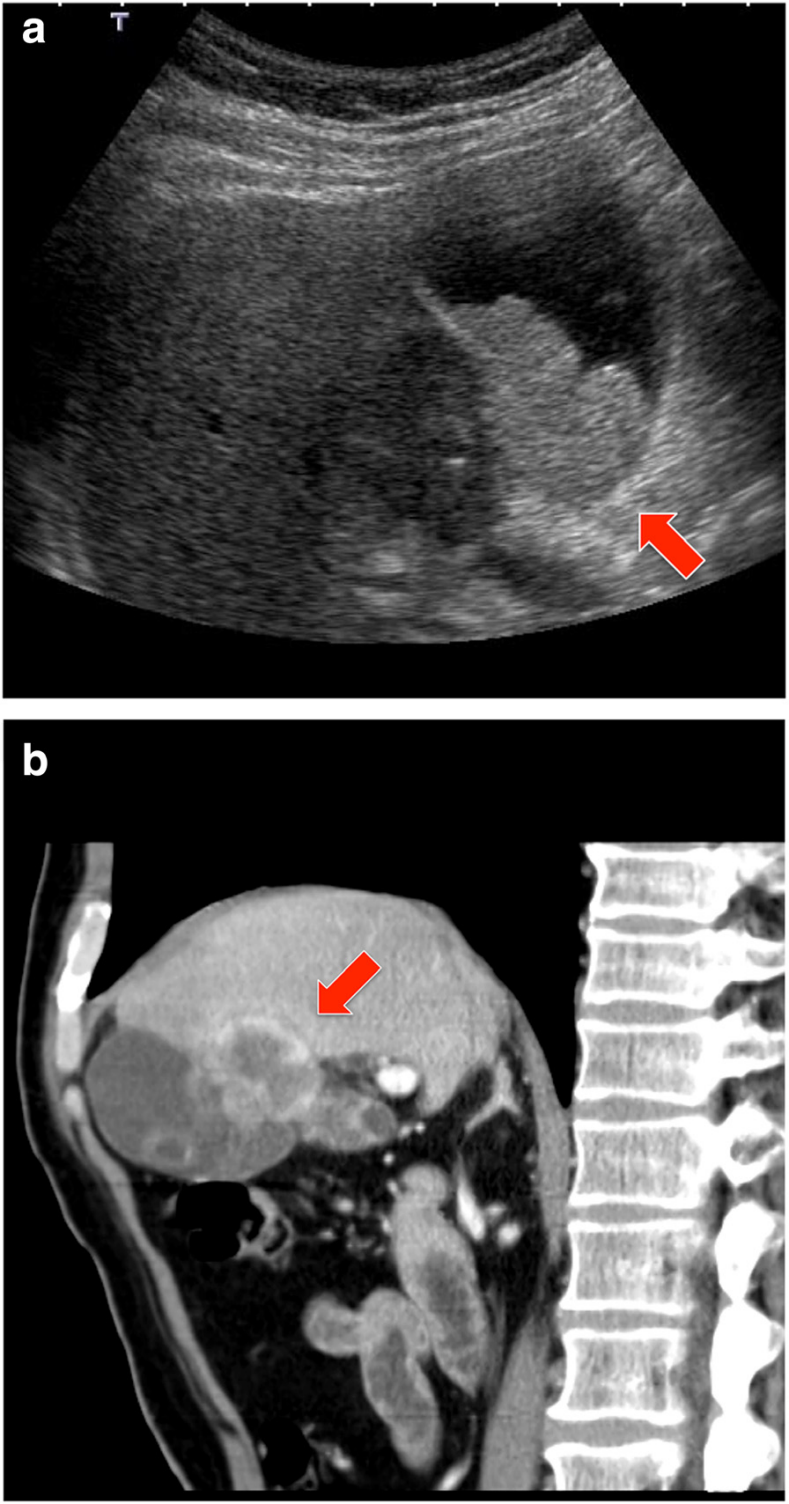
An ultrasonography image (a) and a sagittal section of a computed tomography scan (b) showing the gallbladder carcinoma. The *red arrows* indicate the tumor mass at the neck of the gallbladder, 37 × 30 mm in diameter, involving the liver bed
